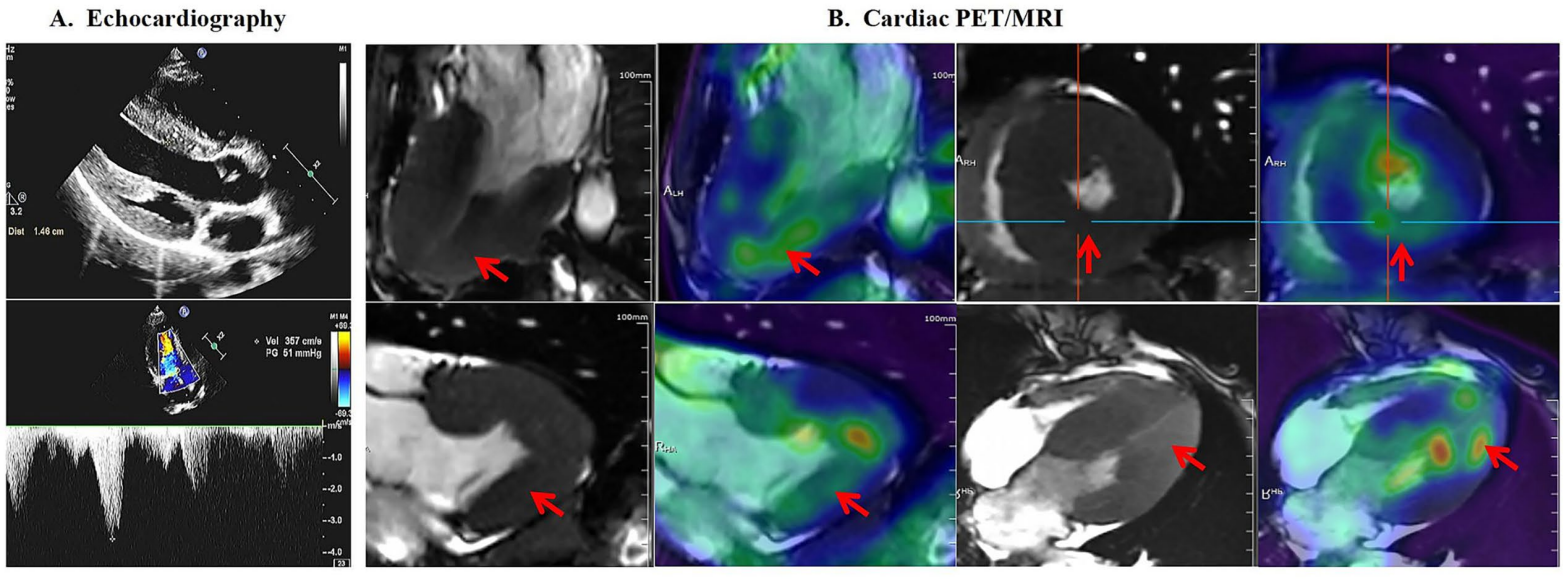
Echocardiography and cardiac positron emission tomography (PET)/magnetic resonance imaging (MRI) of the patient. (A) Echocardiography revealed left ventricular hypertrophy (interventricular septal thickness of 14.6 mm) and left ventricular outflow tract obstruction with a gradient of 51 mmHg after the squatting excitation test. (B) Cardiac PET/MRI revealed a diffusely thickened left ventricular wall and increased metabolism in the inferior and posterior walls (red arrows).
